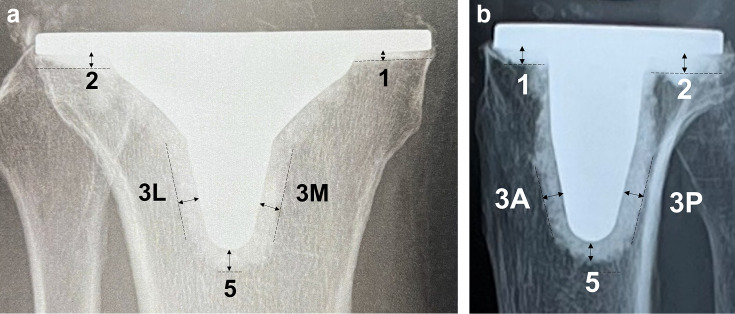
a) Coronal radiographs of implants with zones for documentation of cement mantles. Zone 1: medial baseplate. Zone 2: lateral baseplate. Zone 3: central keel region. Zone 5: the most inferior area of the keel.b) Sagittal radiographs of implants with zones for documentation of cement mantles. Zone 1 of the tibia: anterior baseplate. Zone 2 of the tibia: posterior baseplate. Zone 3 of the tibia: central keel region. Zone 5 of the tibia: the most inferior area of the keel. A, anterior; L, lateral; M, medial; P, posterior.
